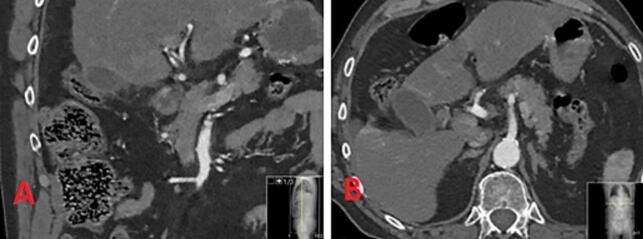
Computed tomography of the chest and the abdomen with an aorta angiography protocol (day 1): A. Frontal view; B. Transverse view.
